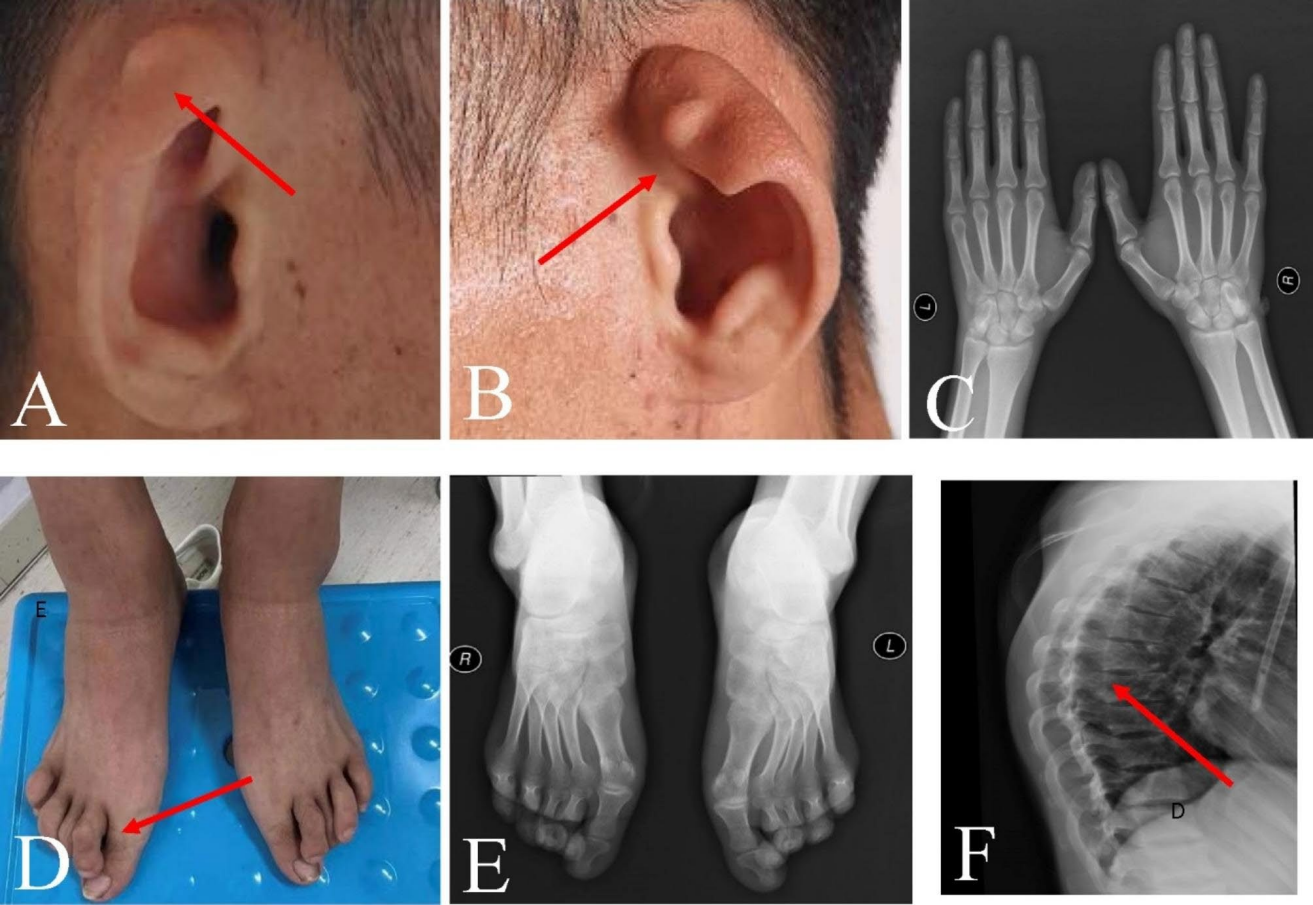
Clinical Features of Patient 1 with Townes-Brocks Syndrome A: Outer ear tag of the right ear (red arrow), B: Outer ear tag of the left ear (red arrow), C: X-ray of both hands, D: toe overlap (red arrow), E: X-ray of both feet, F: Posterior process of thoracic vertebra (red arrow)
